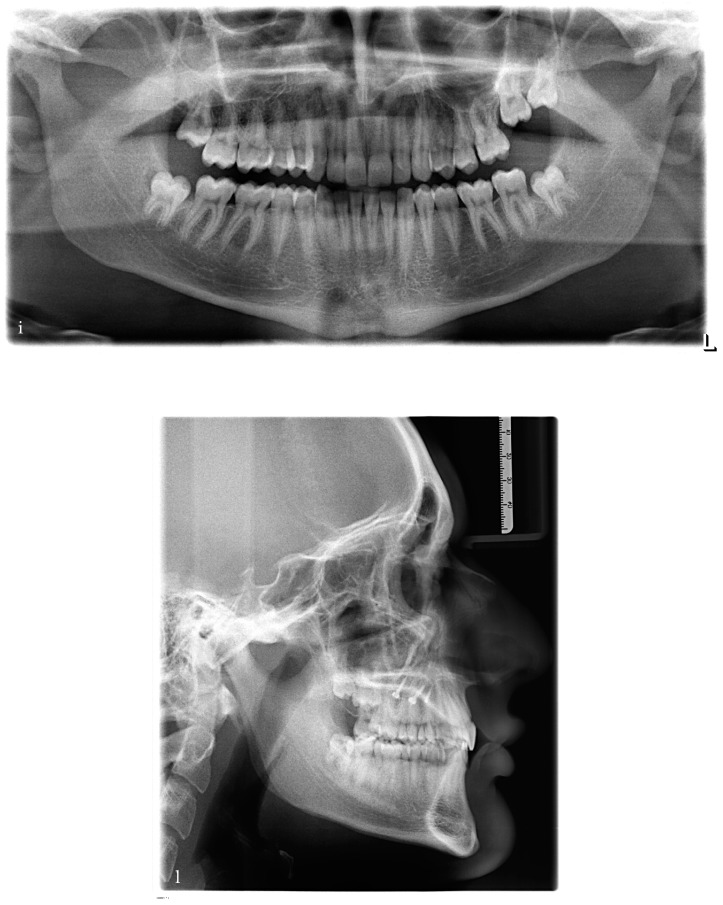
25-year-old male patient with skeletal class I, upper midline deviation, dental Class II subdivision; (a–c) extraoral pre-treatment pictures: lateral and frontal views; (d–f) intraoral pre-treatment pictures: lateral and frontal views; (g,h) occlusal views: upper and lower arch; (i,l): pre-treatment radiographs: panoramic and lateral cephalogram.
